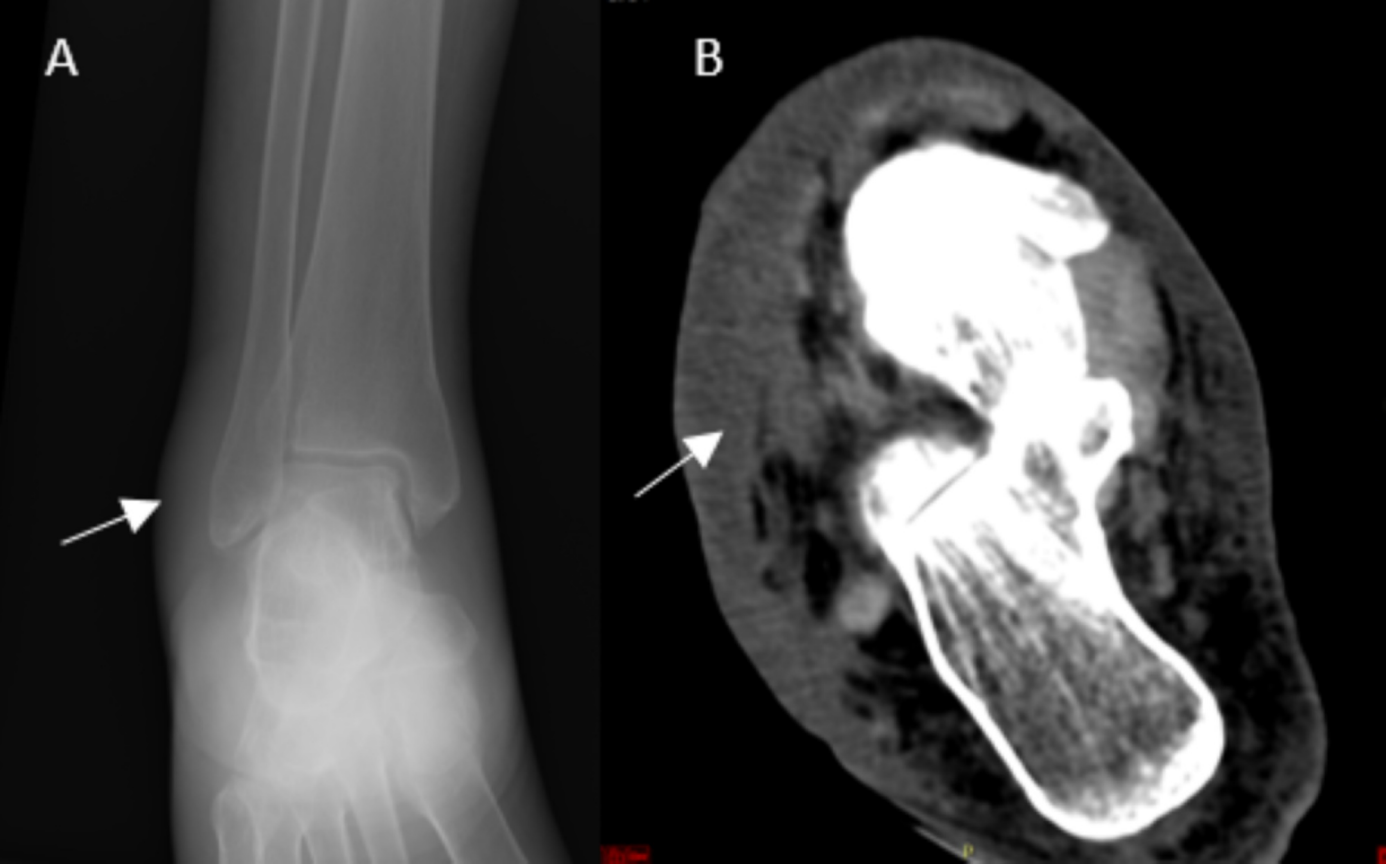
Imaging of the right foot A: X-ray of the right foot. Soft tissue swelling (arrow) around the lateral malleolus. No gas or bony erosions noted. B: CT scan of the right foot. Soft tissue swelling with subcutaneous edema and fluid (arrow) with skin thickening.
